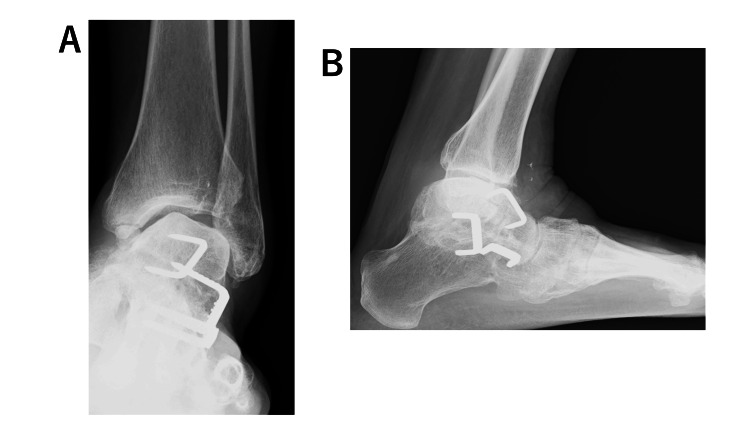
Plain radiographs of the left foot obtained at the first visit. (A) After triple arthrodesis, the plafond was tilted laterally downwards relative to the axis of the tibia, and there was varus displacement of the talus in the ankle mortise. (B) Lateral view showing flattening of the trochlea of the talus.
